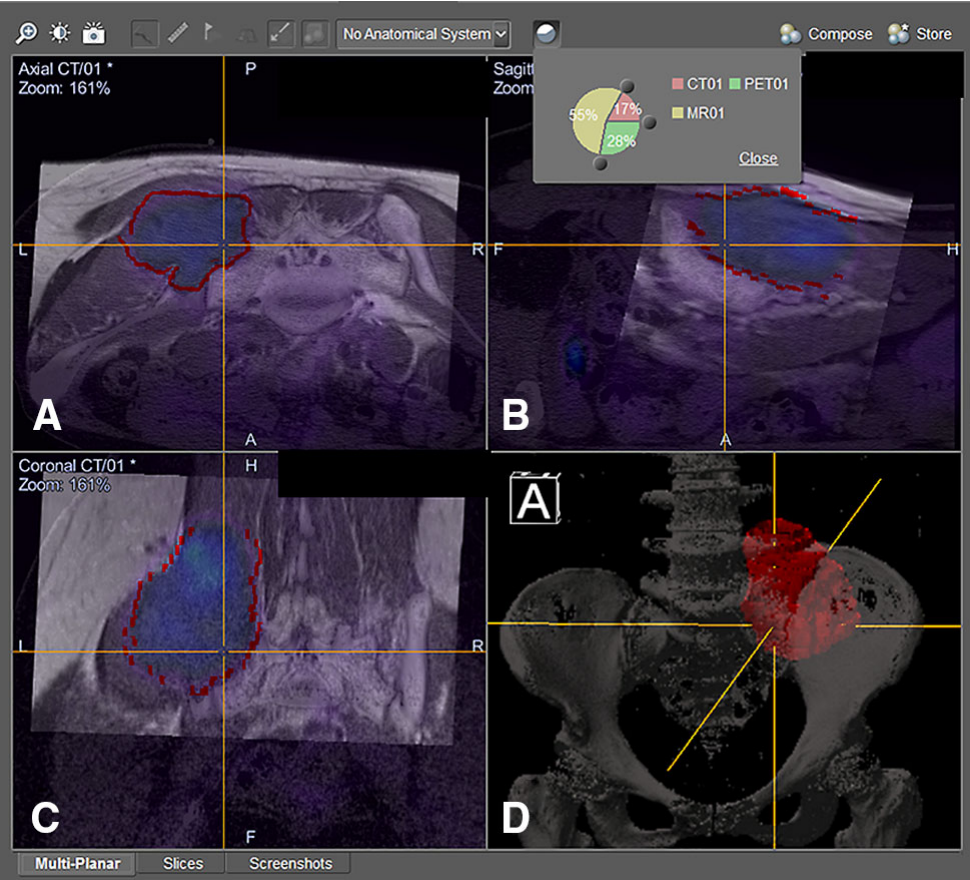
CT/MR/PET fusion images are shown in the navigation display in a patient with a left pelvic tumor involving the posterior superior iliac crest and sacral ala. Wide resection was performed under navigational guidance via a posterior approach, and the left L5 and S1 nerve roots were preserved. Different proportions of image modality could be adjusted on the axial (a), reformatted sagittal (b), and coronal (c) views of the fused images. d A 3D bone tumor model was created after the tumor extent was outlined on the MR images. Surgeons then could accurately define the resection planes after studying the fused 2D images and 3D model
